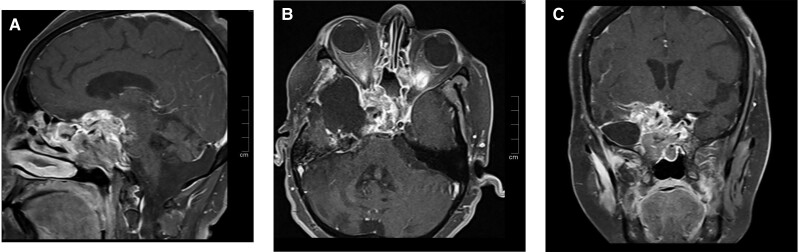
Contrast-enhanced T1 magnetic resonance imaging dynamic pituitary images (A, sagittal; B, axial; C, coronal sections) after 1.5 years of a second session of radiotherapy reveal a significant interval increase in size of heterogeneously enhancing irregular soft tissue in sellar cavity with extension into the right cavernous sinus and parasellar region when compared with previous imaging. Superiorly, it extends in the suprasellar region, causing mass effect on the optic chiasma with encasement of the right prechiasmatic optic nerve and right-sided optic chiasma. Inferiorly, the lesion extends into the sphenoid sinus. Posteriorly, there is interval increase in the lesion involving the clivus and extending into the prepontine and interpeduncular cistern. Anteriorly, mass has reached up to the right orbital apex optic nerve canal, which shows mild interval increase.
